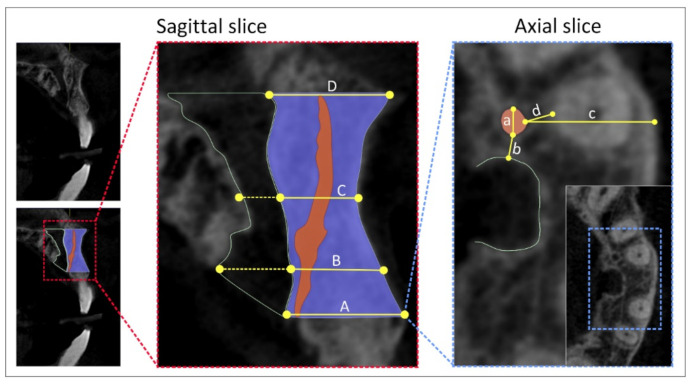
CBCT slices and landmarks of interest. Sagittal cross-section—sagittal CBCT slice (left, upper); slice with marked field of interest (left, bellow); selected landmarks for analyses (right)—(A) the distance between the buccal border of incisive foramen and facial aspect of the buccal bone wall, (B) the distance between the buccal wall of the nasopalatine canal and facial aspect of the buccal bone wall using a horizontal line from the palatal border of the incisive foramen, (C) the distance between the buccal border at the midpoint level of NPC length and facial aspect of the buccal bone wall, (D) the distance between the buccal border of nasal foramen and facial aspect of the buccal bone wall; Axial cross-section—axial CBCT slice (right); (a) the diameter of AC, (b) the distance between AC and NPC, (c) the distance between AC and facial aspect of the buccal bone wall, (d) the distance between AC and central incisor root.
